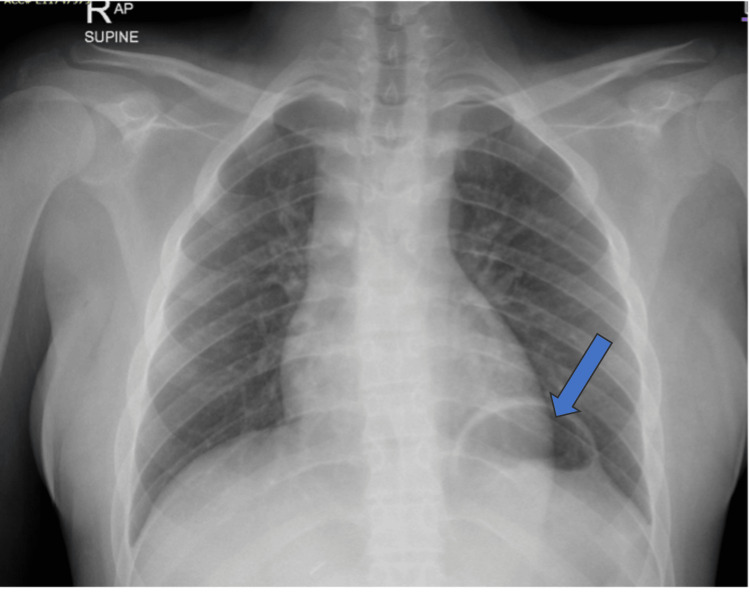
Chest radiograph on admission demonstrating clear lung fields but focal bulging beneath the left hemidiaphragm (arrow), initially interpreted as possible eventration.
